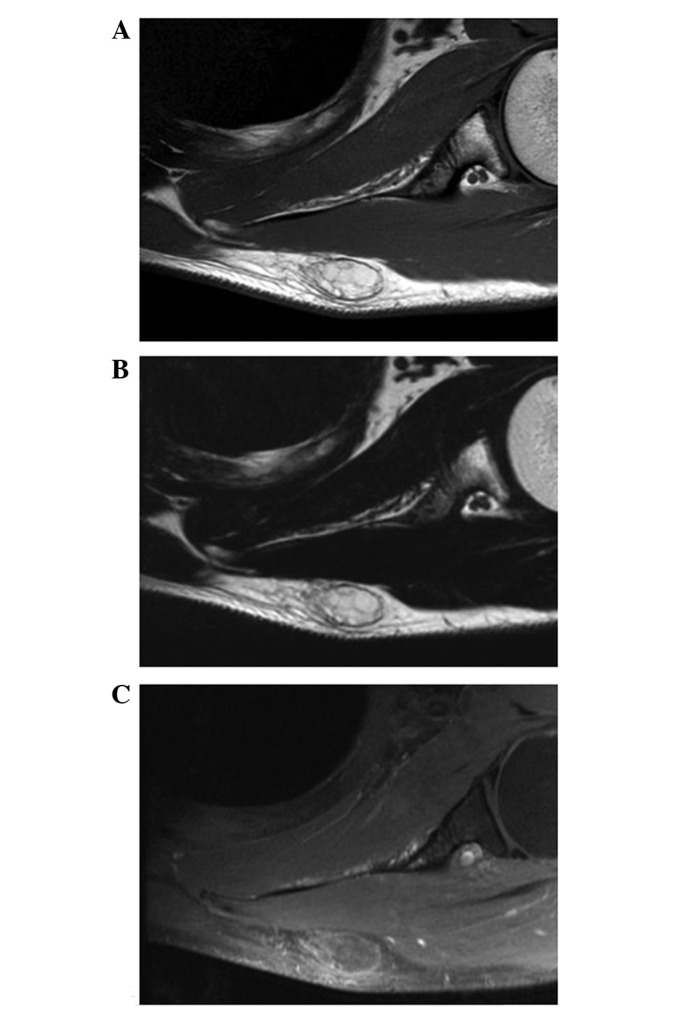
Axial magnetic resonance imaging (MRI) of an osteochondrolipoma in the left scapular region. (A) T1-weighted sequence showing the mass with an almost homogeneous high signal intensity. (B) T2-weighted sequence showing the mass with almost homogeneous high signal intensity. (C) Contrast-enhanced fat-suppressed T1-weighted sequence showing a faint peripheral and septal enhancement of the mass.
